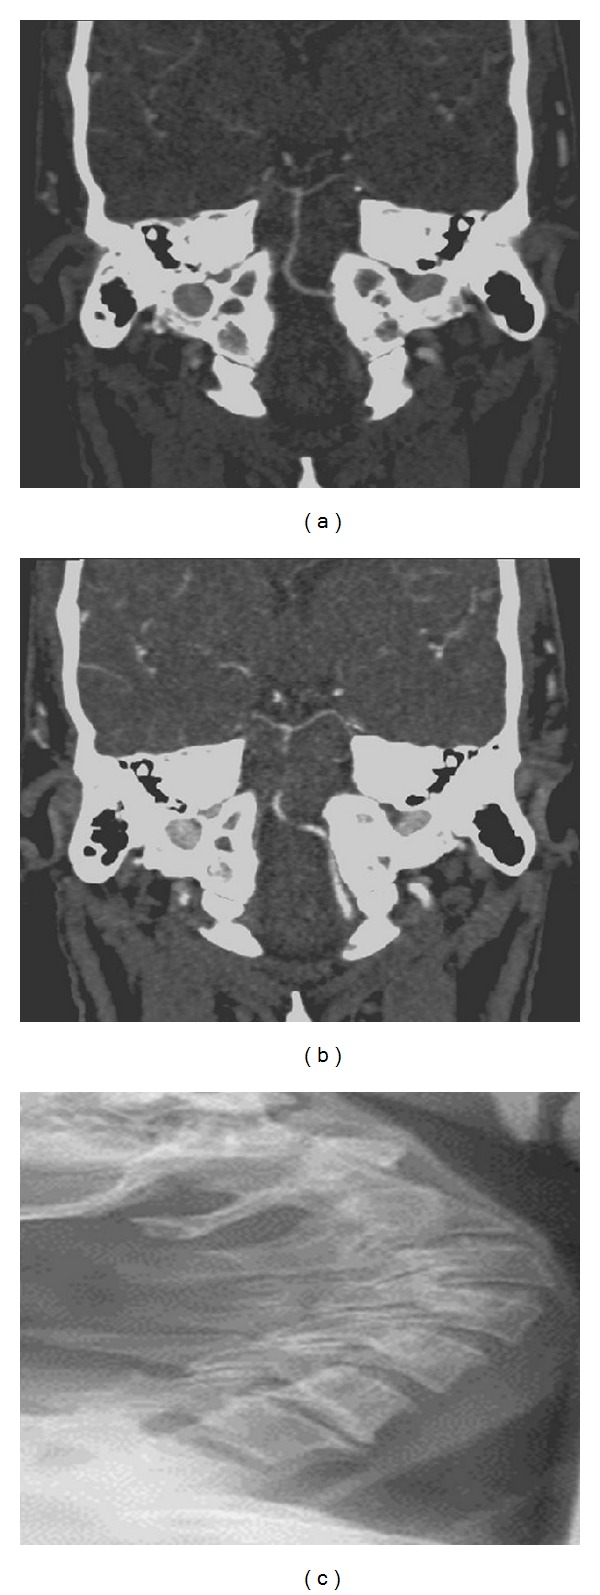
(a) and (b) CTA indicates dominant left vertebral artery and hypoplastic right vertebral artery with minimal contribution in formation of the small basilar artery. (c) Lateral cervical spine X-ray in extension showing diffused degenerative changes with no evidence of cervical instability.
